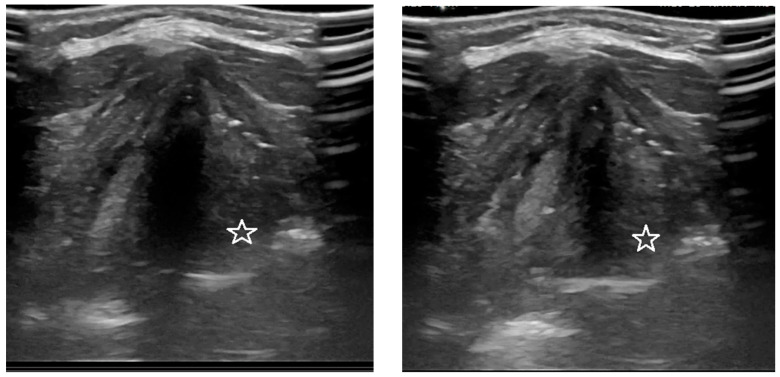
Asymmetry of the glottis during breathing on ultrasound. Reduced mobility and adduction of the left vocal fold (asterisk).
